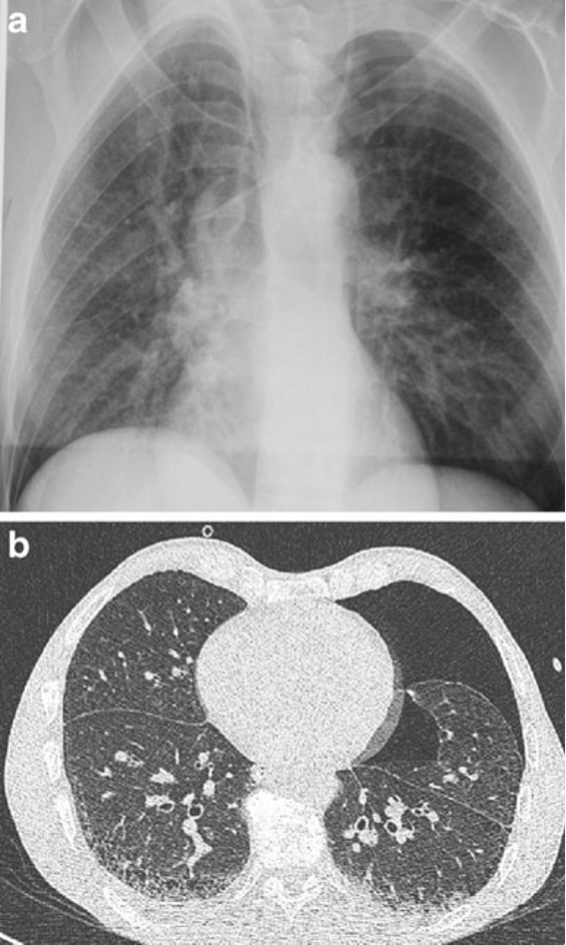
a, bPneumothorax: discrepancy between the supine chest radiograph and MDCT. aChest radiograph reveals slightly increased transparency of the left lung and thin linear translucency contouring the left border of the lower mediastinum. bMDCT shows significant pneumothorax in the ventral part of the left pleural cavity
